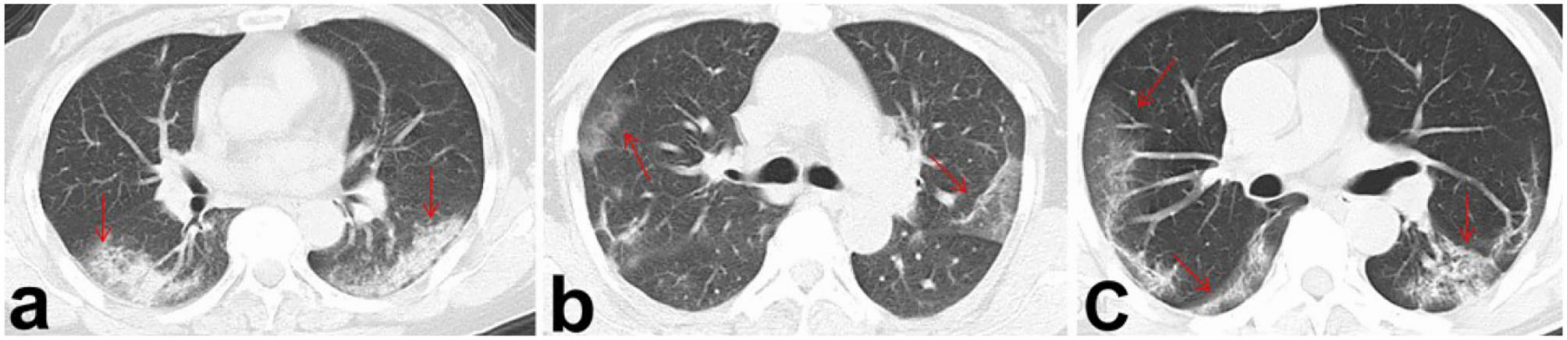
Viral pneumonia of pulmonary CT imaging in three patients infected with the Alpha strain. The lesions were marked by red arrows in panels (a–c). Bilateral involvement and multiple lesions located in peripheral zones of the lungs were found.
